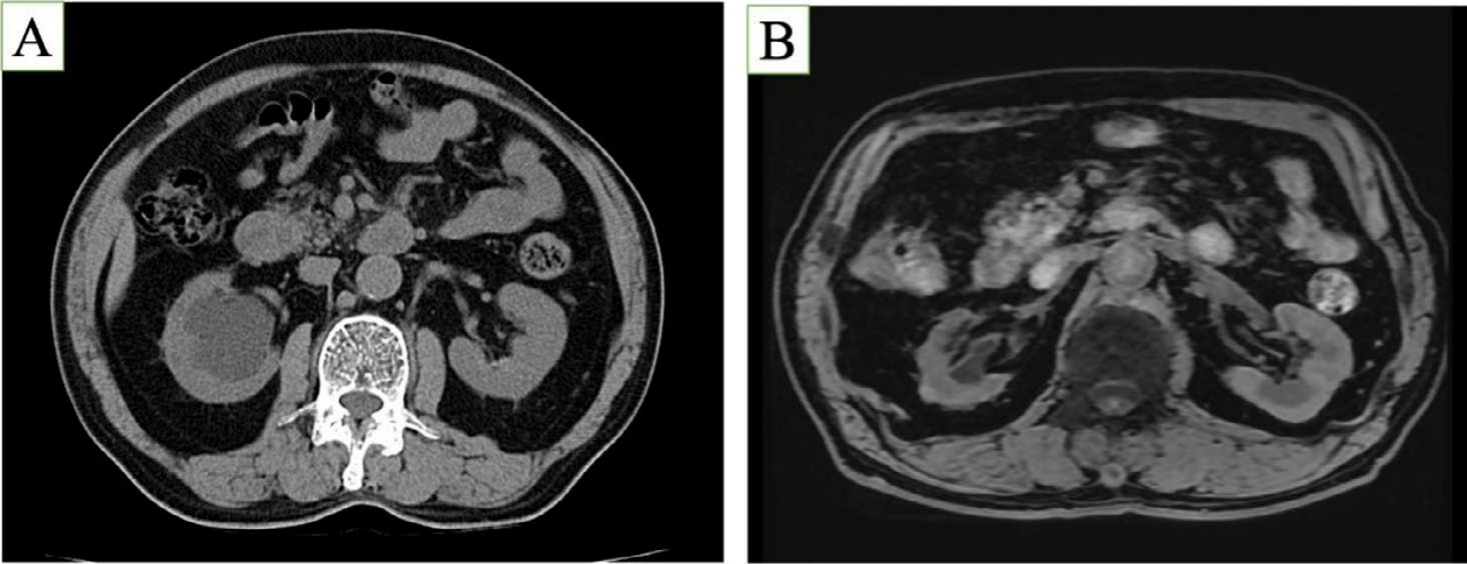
(A) Hydronephrosis before surgery. (B) On Day 226, magnetic resonance imaging indicated improved hydronephrosis.
